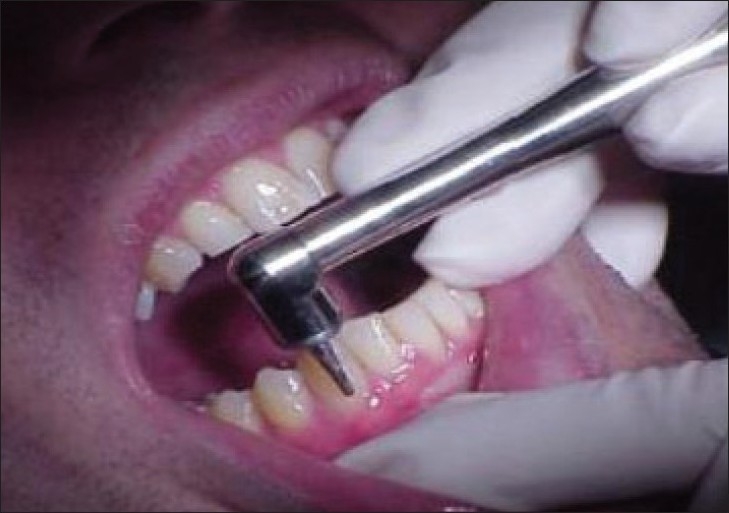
Ultrasonographic periodontal probe in use; (as.wm.edu/Faculty/Hinders/NDE-Projects.html)
